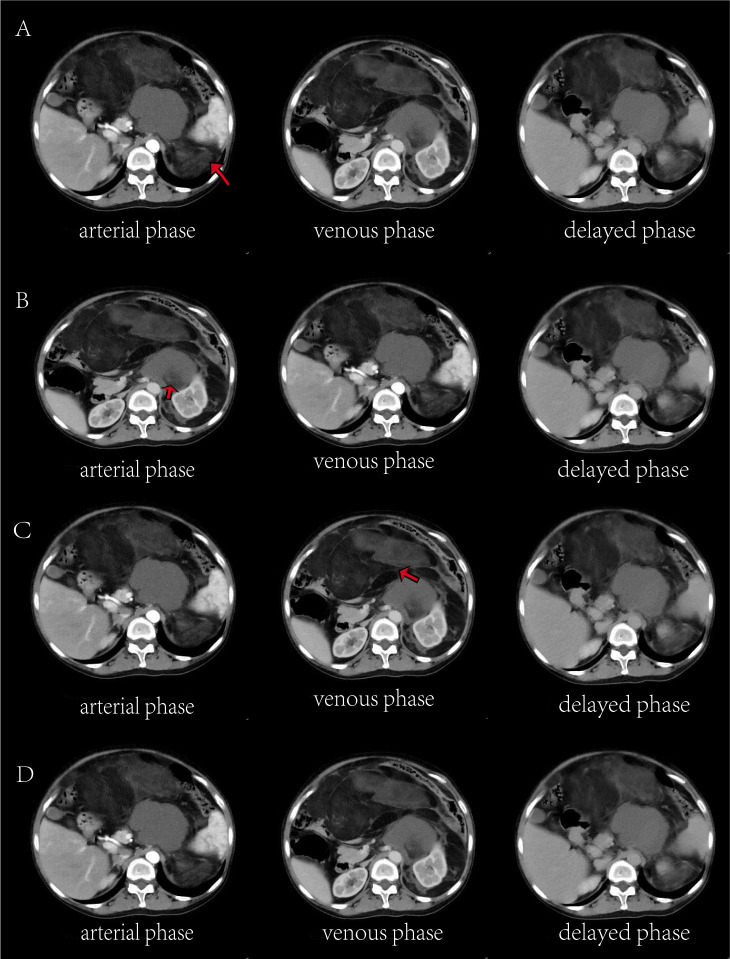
Axial CT images in the arterial (A), venous (B), and delayed (C) phases demonstrate four discrete lesions. (A) Arrow indicates a lesion containing hypodense fatty components. (B) Arrow denotes the left renal artery. (C) Arrow highlights a lesion with hyperdense solid components.
